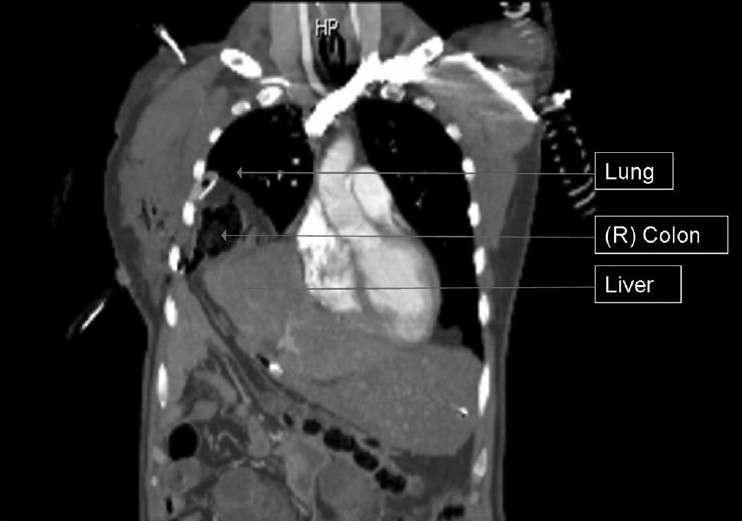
Coronal computed tomography scan demonstrating hernia of the right diaphragm with right colon and right liver lobe in the right chest cavity
